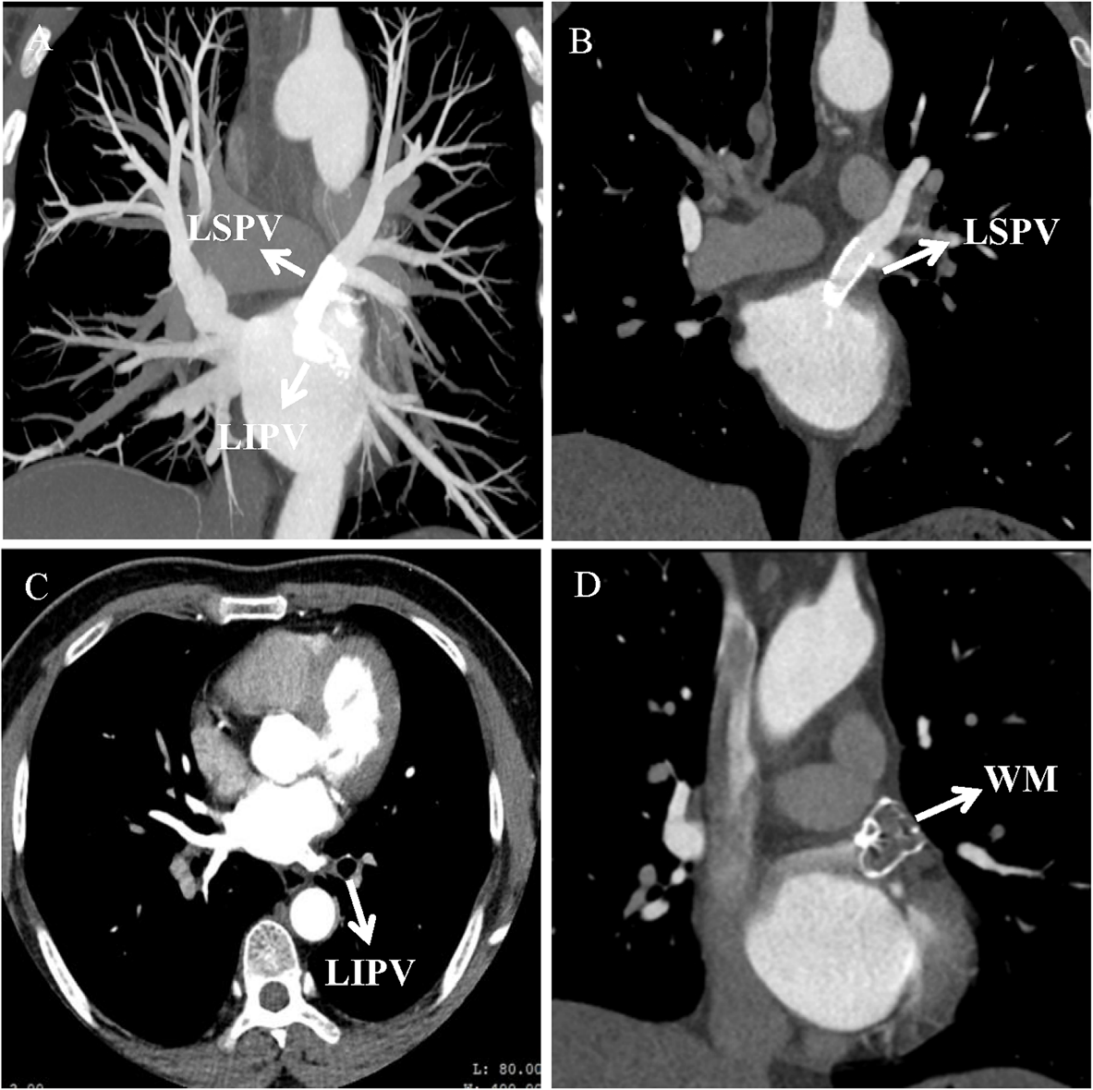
a Restored pulmonary blood flow. b, c No in-stent restenosis. d No device-related thrombus in CT scan at 6 months post-procedure
